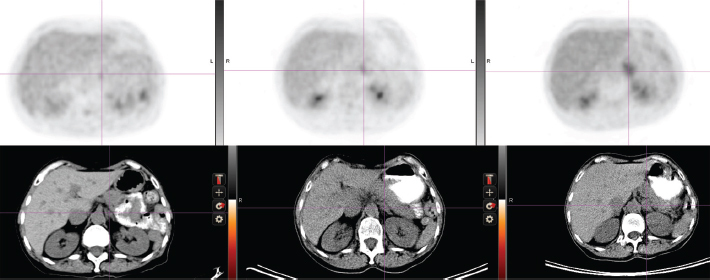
Abdominal cross-section showing left adrenal mass and FDG uptake (upper panel). See text for time points.
